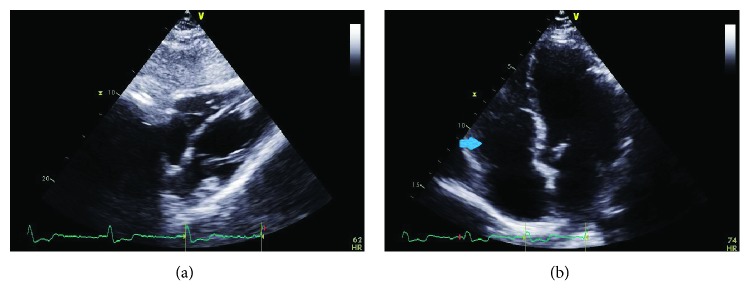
(a) Subcostal view on transthoracic echocardiogram two years postprocedure without evidence of recurrence of RV myxoma. (b) Apical view from the same study (arrow: right ventricle).
